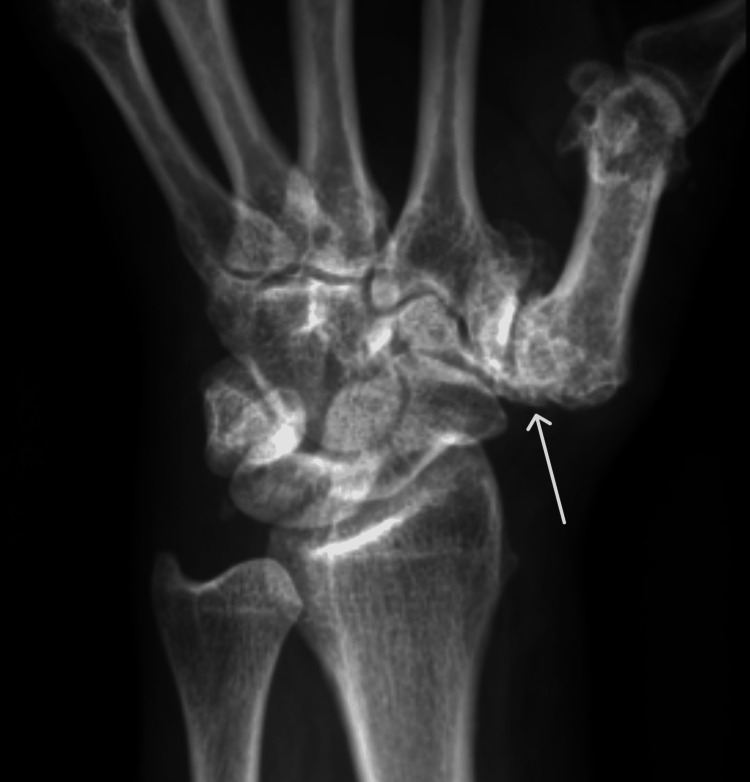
Oblique radiograph of the left wrist demonstrating advanced neuropathic arthropathic changes, including joint space obliteration, subchondral sclerosis, cystic changes, bony fragmentation, carpal disorganization, and volar subluxation of the first metacarpal, consistent with ipsilateral wrist and first carpometacarpal joint involvement secondary to syringomyelia. The abnormalities are indicated by white arrows.
